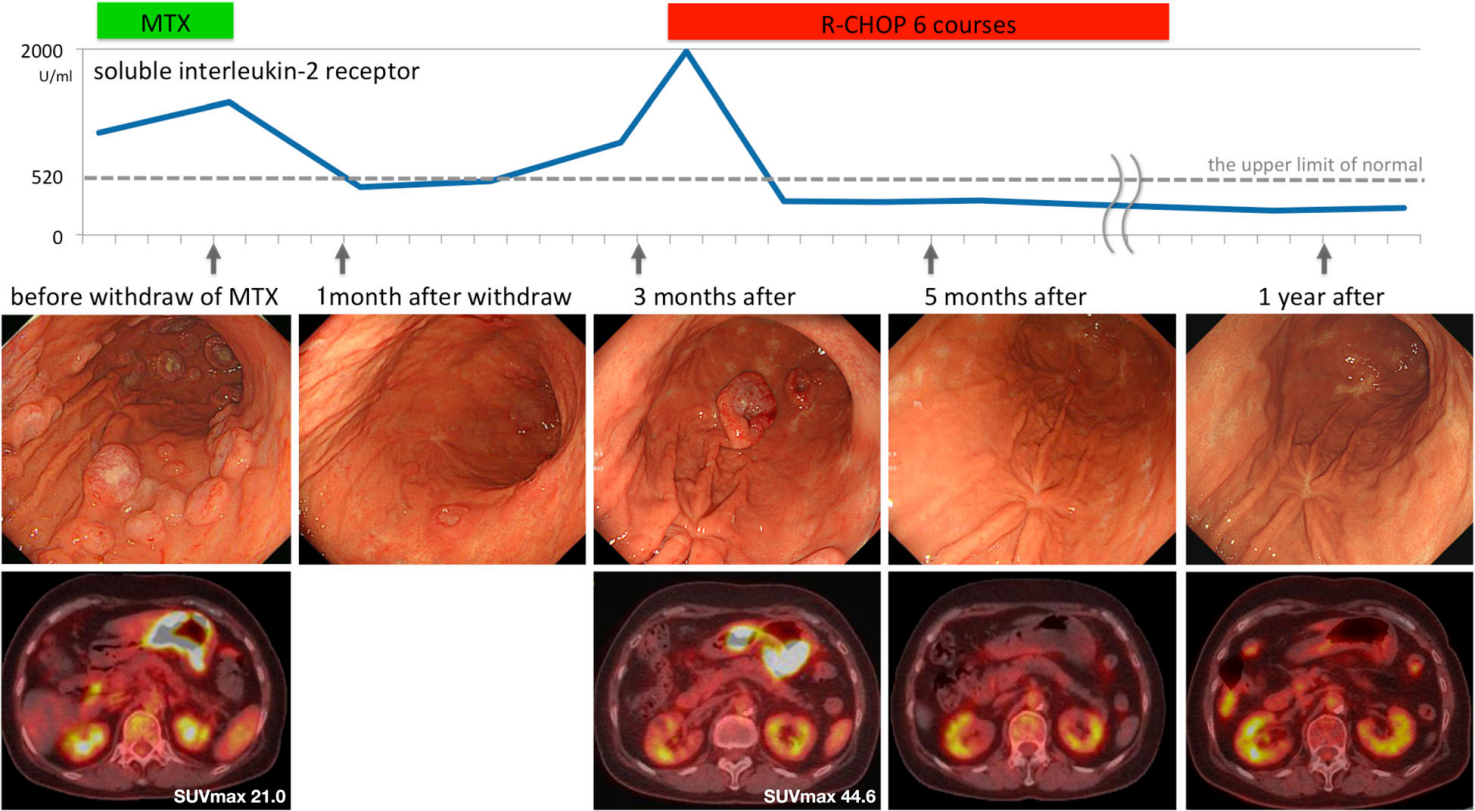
Clinical causes: We observed changes in MTX-LPD symptoms in the stomach and duodenum with regard to the levels of sIL-2R, and EGD and PET-CT findings
